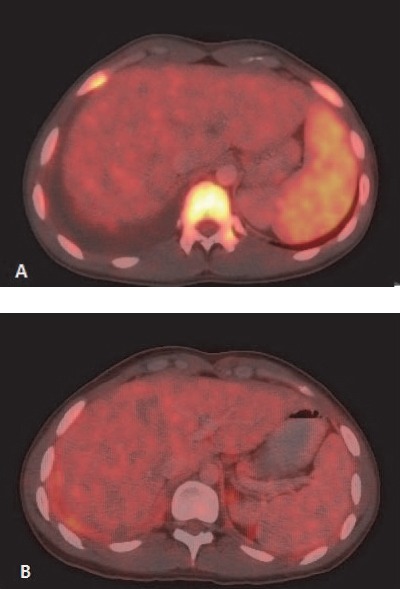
Diffuse involvement of spleen in a case of NHL is seen (A). Note greater FDG avidity of spleen as compared to liver. Post therapy scan shows complete metabolic response (B)
